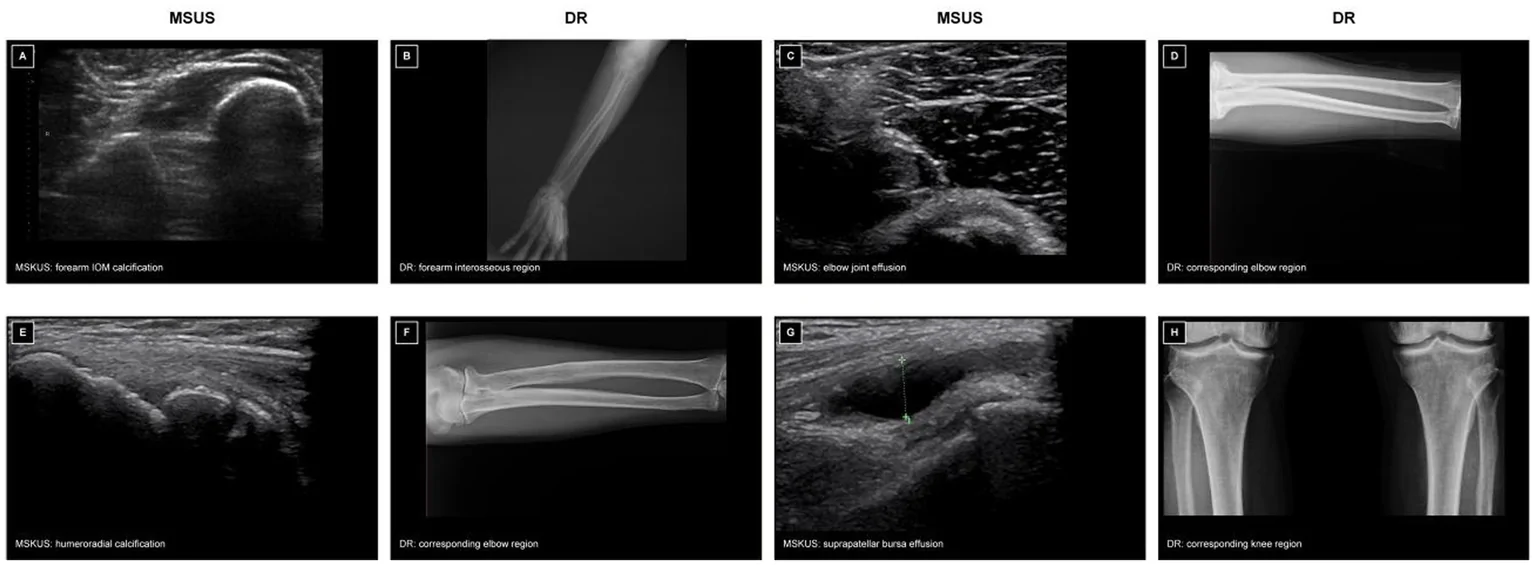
Representative musculoskeletal ultrasound and digital radiography findings in endemic skeletal fluorosis. (A,B) Musculoskeletal ultrasound (MSKUS) image showing calcification of the forearm interosseous membrane (IOM), with the corresponding digital radiography (DR) image of the forearm region. (C,D) MSKUS image showing elbow joint effusion, with the corresponding DR image of the elbow region. (E,F) MSKUS image showing humeroradial joint calcification, with the corresponding DR image of the elbow region. (G,H) MSKUS image showing suprapatellar bursa effusion, with the corresponding DR image of the knee region. MSKUS, musculoskeletal ultrasound; DR, digital radiography; IOM, interosseous membrane.
